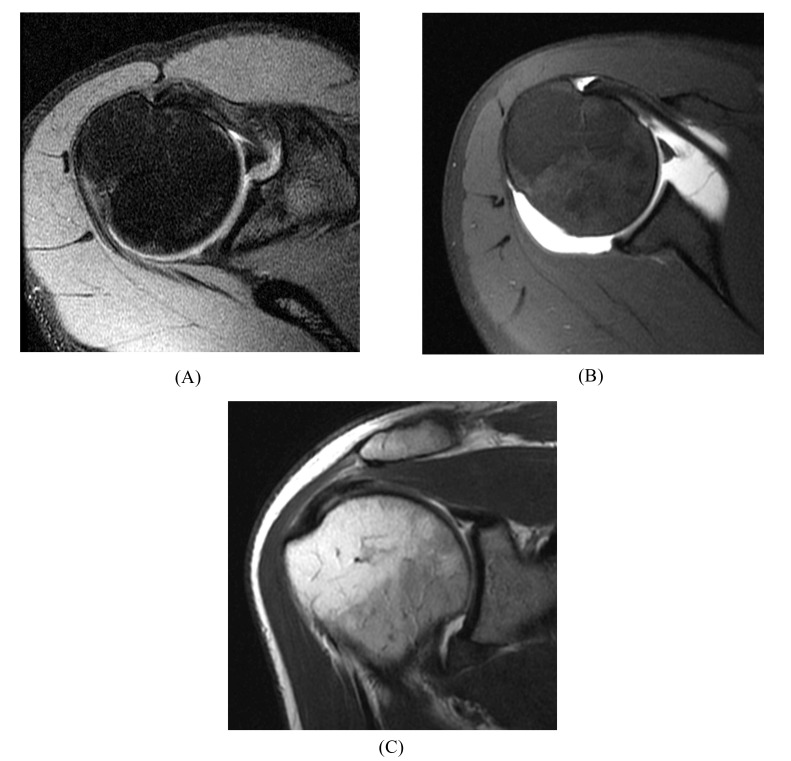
Buford complex. (A) Axial fat-suppressed T2-weighted image of a 39 year-old male athlete with an absent anterosuperior labrum and a thickened, cord-like middle glenohumeral ligament. (B) Axial fat-suppressed T1-weighted MR arthrogram image in a 44 year-old orthopaedist, a female athlete with a Buford complex. (C) Coronal T1-weighted MR arthrogram image in the same shoulder shows a superior labral tear.
